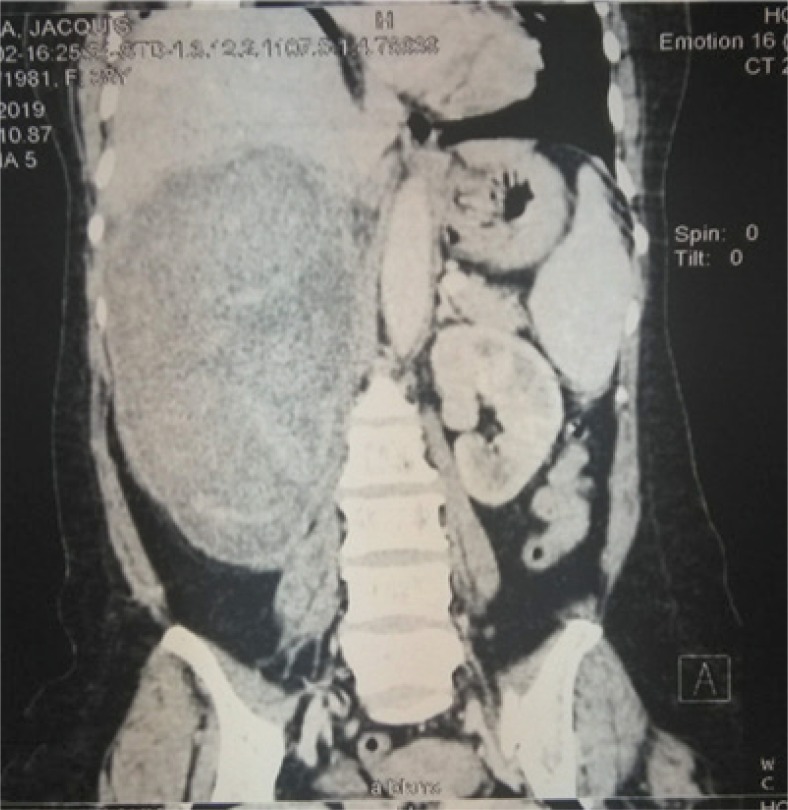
A contrast coronal CT-scan showing a hyper vascularized right T4 renal tumor found to have affected the renal vein, IVC, and inferior margin of the liver.
